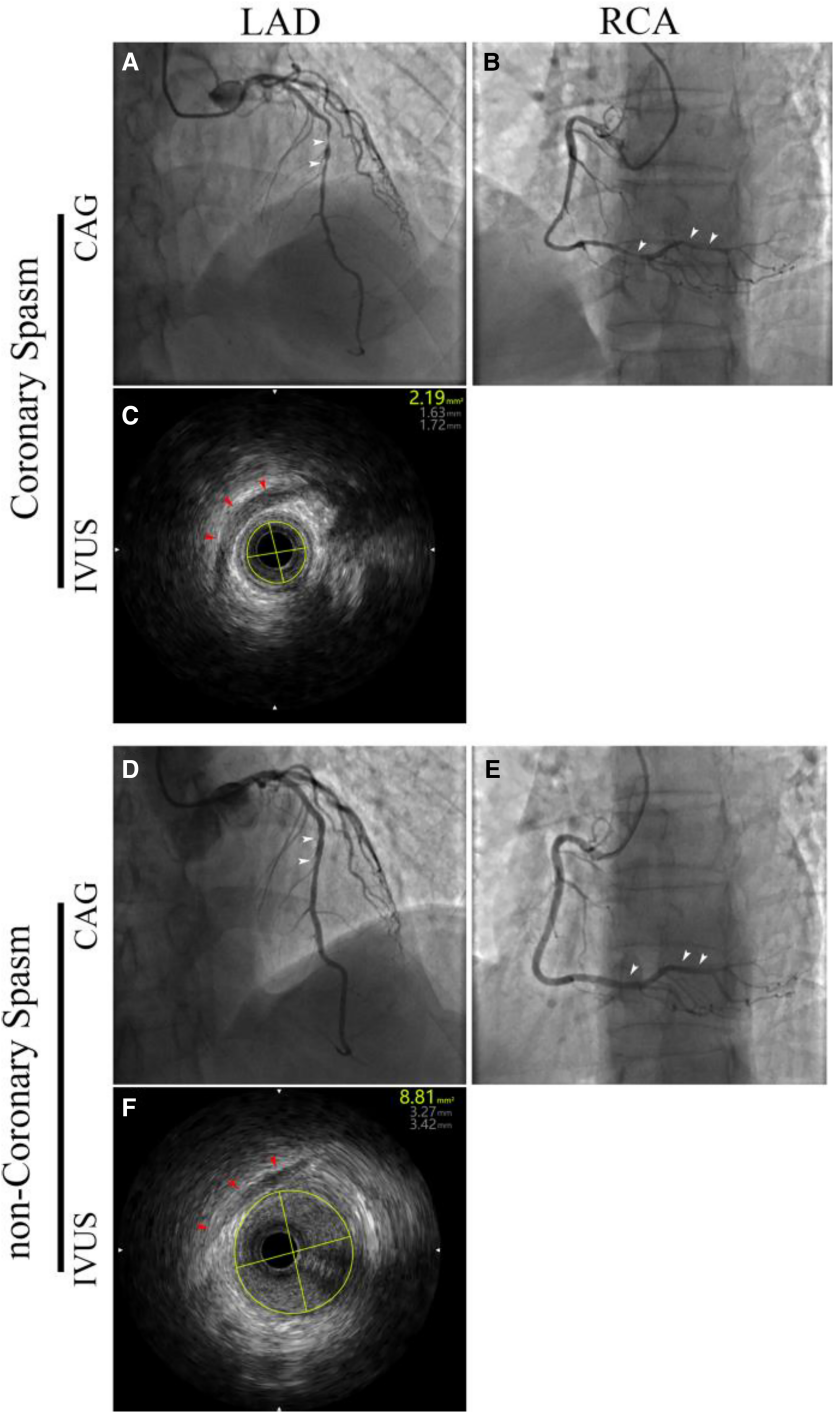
Emergency coronary angiography (CAG) and intravascular ultrasound (IVUS) were conducted in response to a recurrence of chest pain one day ago. During coronary spasm: CAG showed (A) the entire segment of the left anterior descending (LAD) artery was slender, with mid-segment narrowing of 95%–99% (white arrow), and (B) the entire segment of the right coronary artery (RCA) was slender, with part of the segment narrowing 95%–99% (white arrow); (C) myocardial bridging (red arrow), with the minimum luminal area of the LAD at 2.19 mm^2^. During non-coronary spasm: (D) the entire segment of the LAD was significantly dilated compared to before, with segmental narrowing resolved (white arrow); (E) the entire segment of the RCA was significantly dilated compared to before, with segmental narrowing disappeared (white arrow); (F) follow-up IVUS showed that the luminal area at the initially narrowest point had recovered to 8.81 mm^2^.
